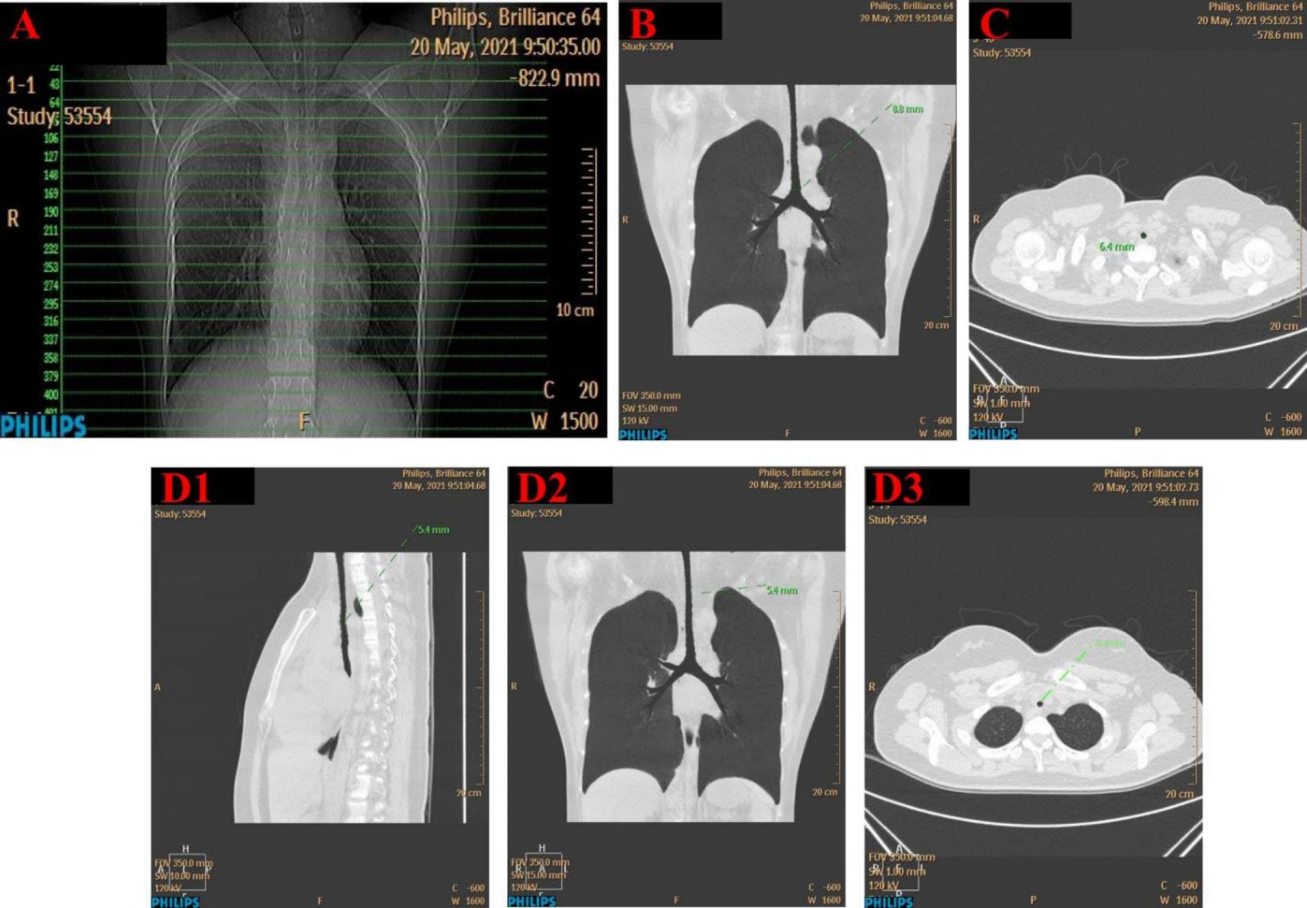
Coronal and sagittal measurements on CT and three-dimensional image reconstruction shows that the whole segment of the trachea and bilateral bronchi were all narrow. The minimum diameter of the trachea was only 5.4 mm. The maximum was 8.8 mm. The airway diameter in normal adults is approximately 15 mm ~ 20 mm
